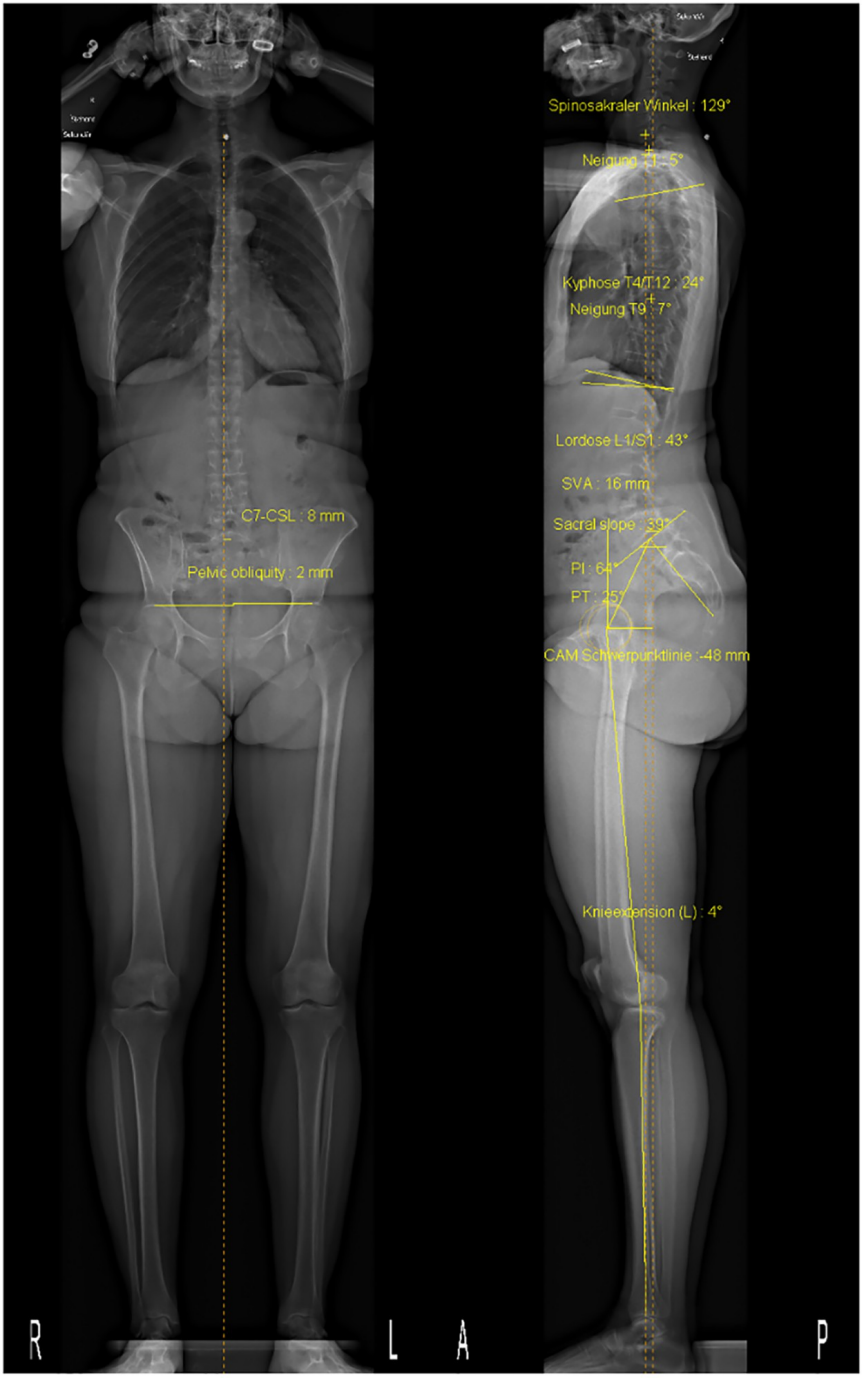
Example of an EOS radiography (left: frontal plane; right: sagittal plane) with measures provided by the sterEOS software.
